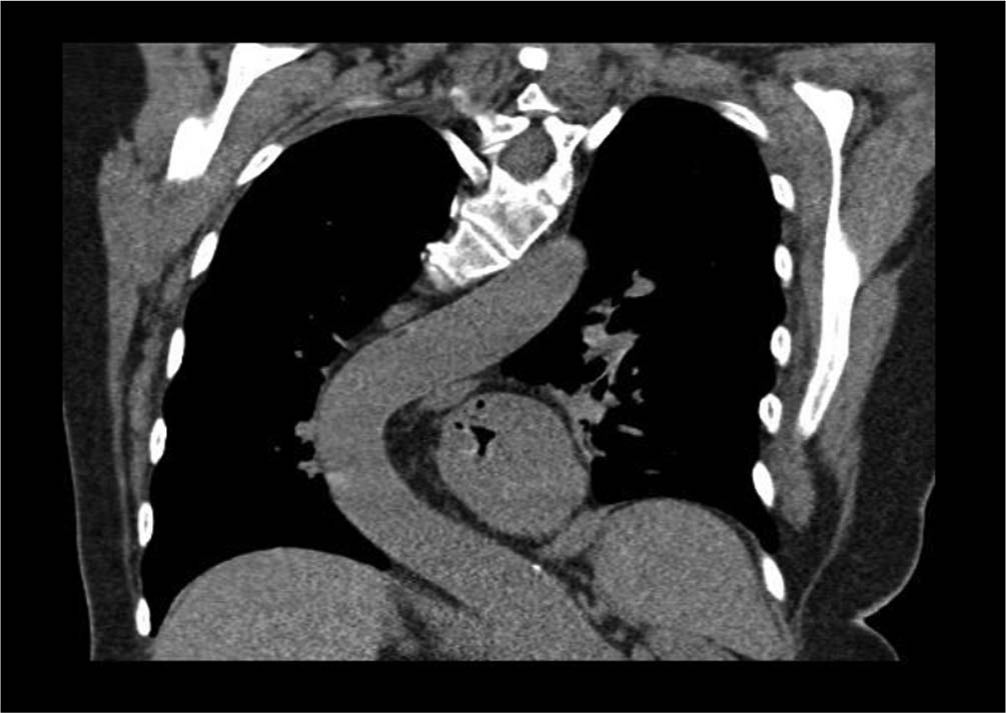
Coronal CT showing curvature of the thoracic aorta tortuosity compressing the distal esophagus and causing chronic left lower lobe segmental atelectasis.
